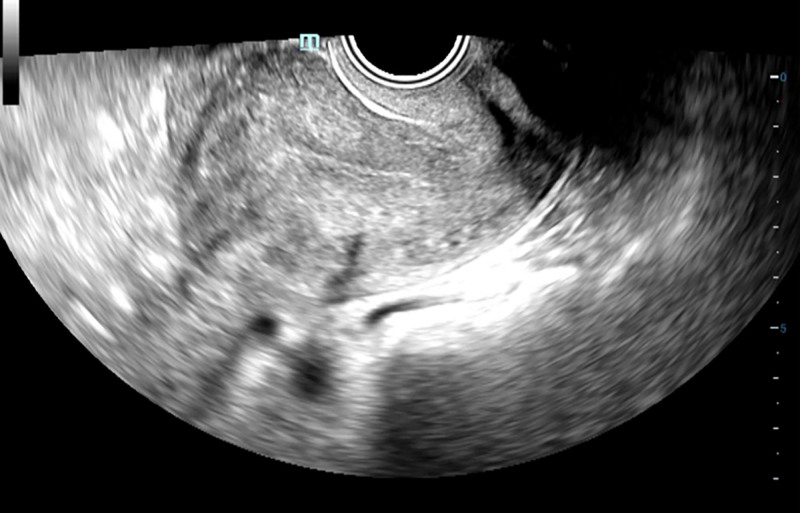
Gynecological ultrasound image of Patient 2.
